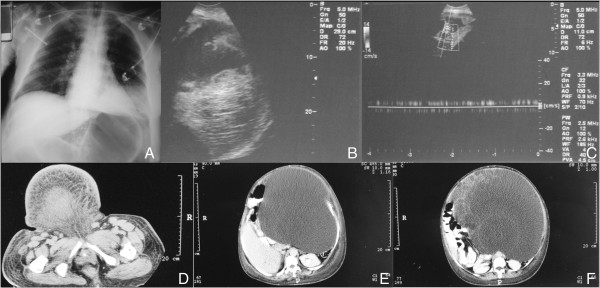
Imaging studies of the giant mucinous cystadenocarcinoma of the ovary. (A) Chest radiograph showing an upward compression of the diaphragm. (B) Ultrasound images of the heterogeneous mass, which occupies the entire abdominal cavity. (C) Abdominal ultrasound (US) showing normal liver, spleen and kidneys. (D-F) Contrasted computed tomography (CT) scan revealing an extra-large mass with well-defined limits, and heterogeneous coefficient of attenuation, which was predominantly liquid. The mass appeared to extend from the right adnexal area up to the diaphragmatic region, displacing the abdominal contents.
